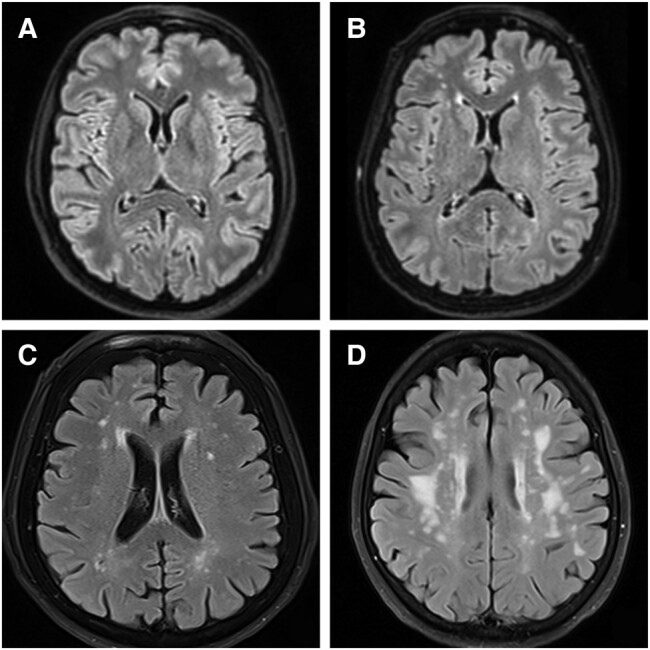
Brain MRI showing different Fazekas classification types. (A) Fazekas 0: no white matter hyperintensities. (B) Fazekas 1: punctate hyperintensities. (C) Fazekas 2: early confluent hyperintensities. (D) Fazekas 3: confluent hyperintensities with early ventricular involvement.
